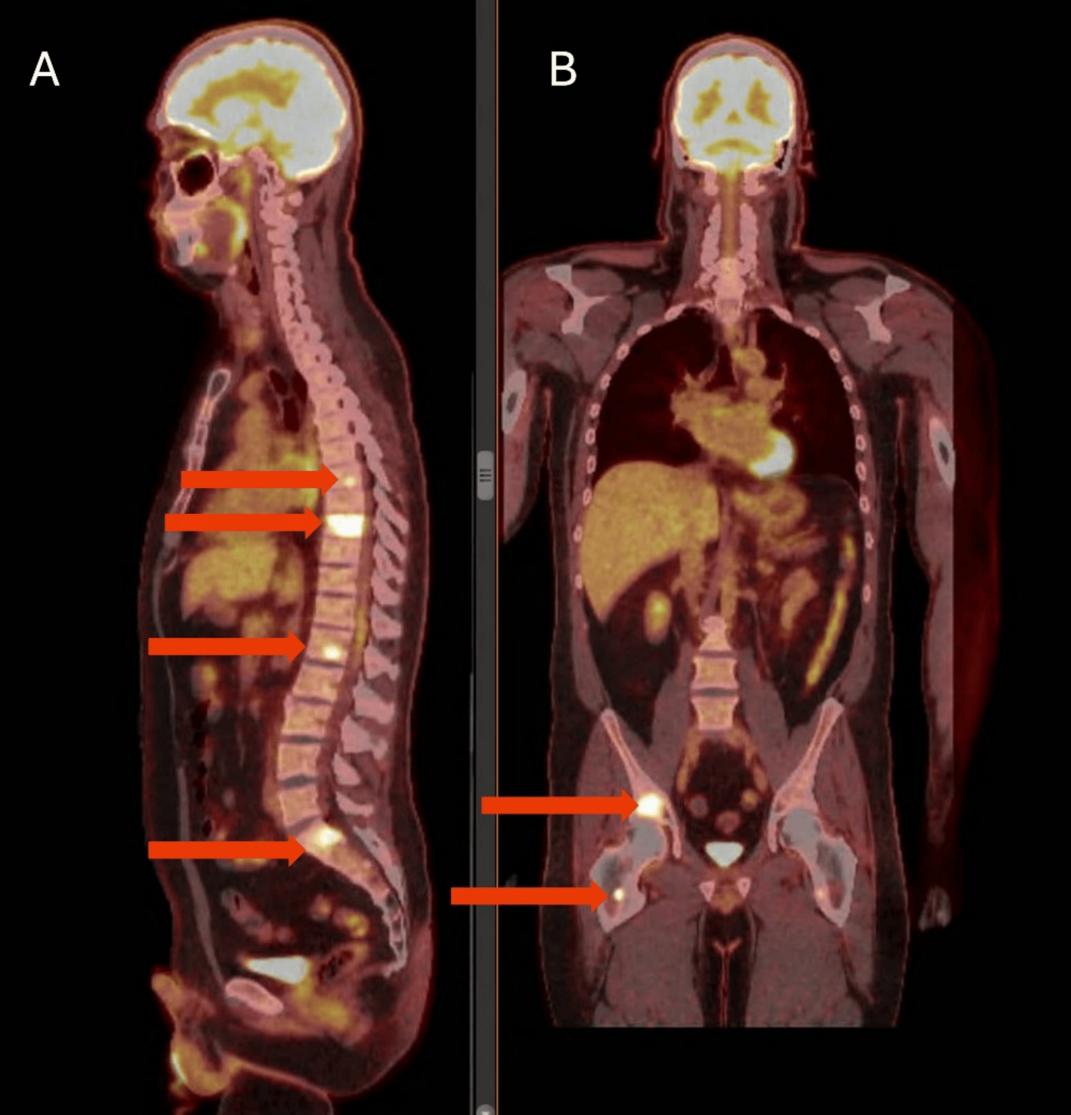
Whole-body PET/CT F-18 fluorodeoxyglucose (FDG): (A) sagittal and (B) coronal view (Summer 2024): Multiple hypermetabolic osseous lesions in the axial and appendicular skeleton concerning for metastatic disease. The red arrow shows PET FDG-avid lesion.
